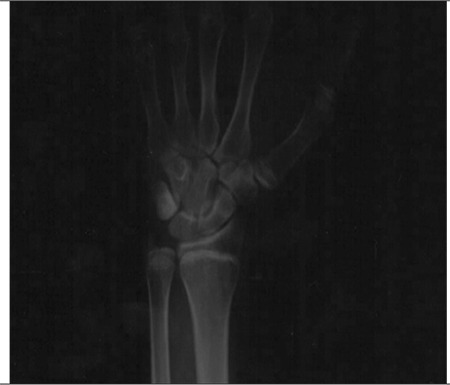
Plain bone radiography of the left wrist
